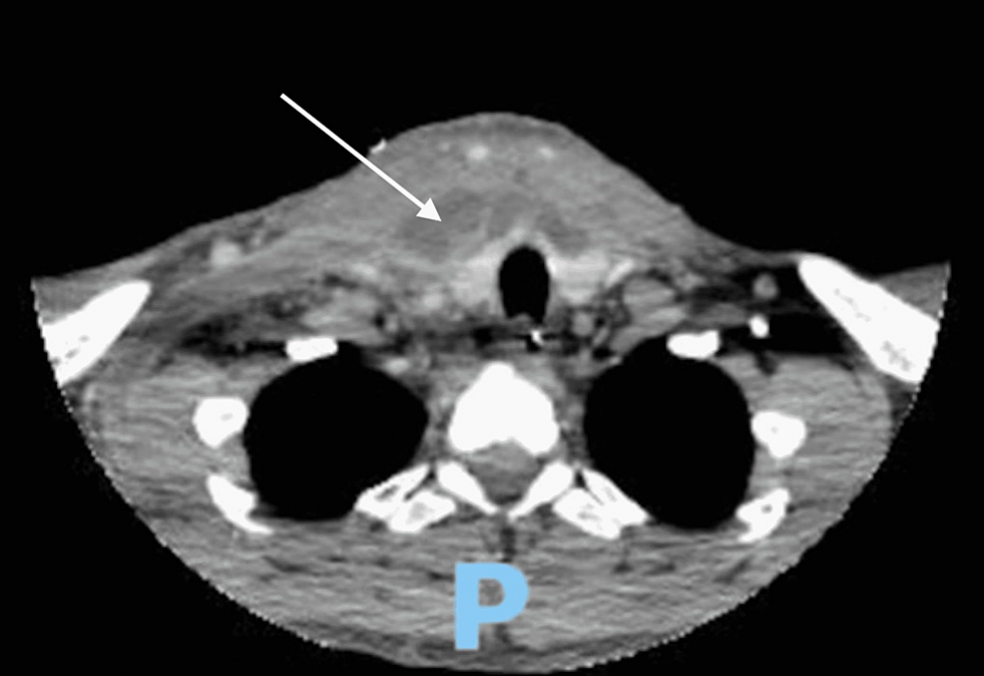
Axial view of CT scan on hospital day 5 Axial view of CT scan on hospital day 5 showed multiple fluid collections (white arrow) extending from the right submandibular space into the right carotid space, right prevertebral space, and the right retropharyngeal space. In addition, the chest CT showed communication of the right neck collections crossing midline and below the thyroid into the retropharyngeal space and inferiorly into the anterior and middle mediastinum compartments.
